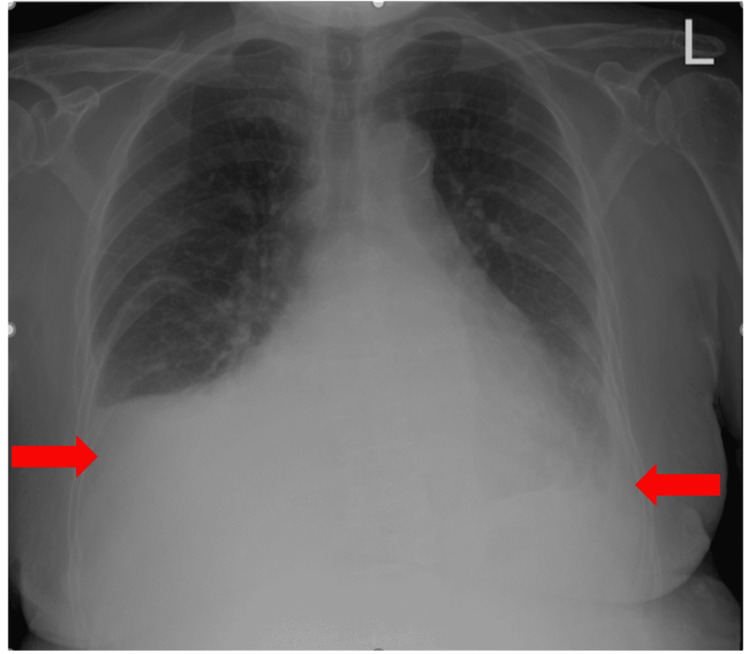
Chest x-ray depicting large right and small left pleural effusion.
